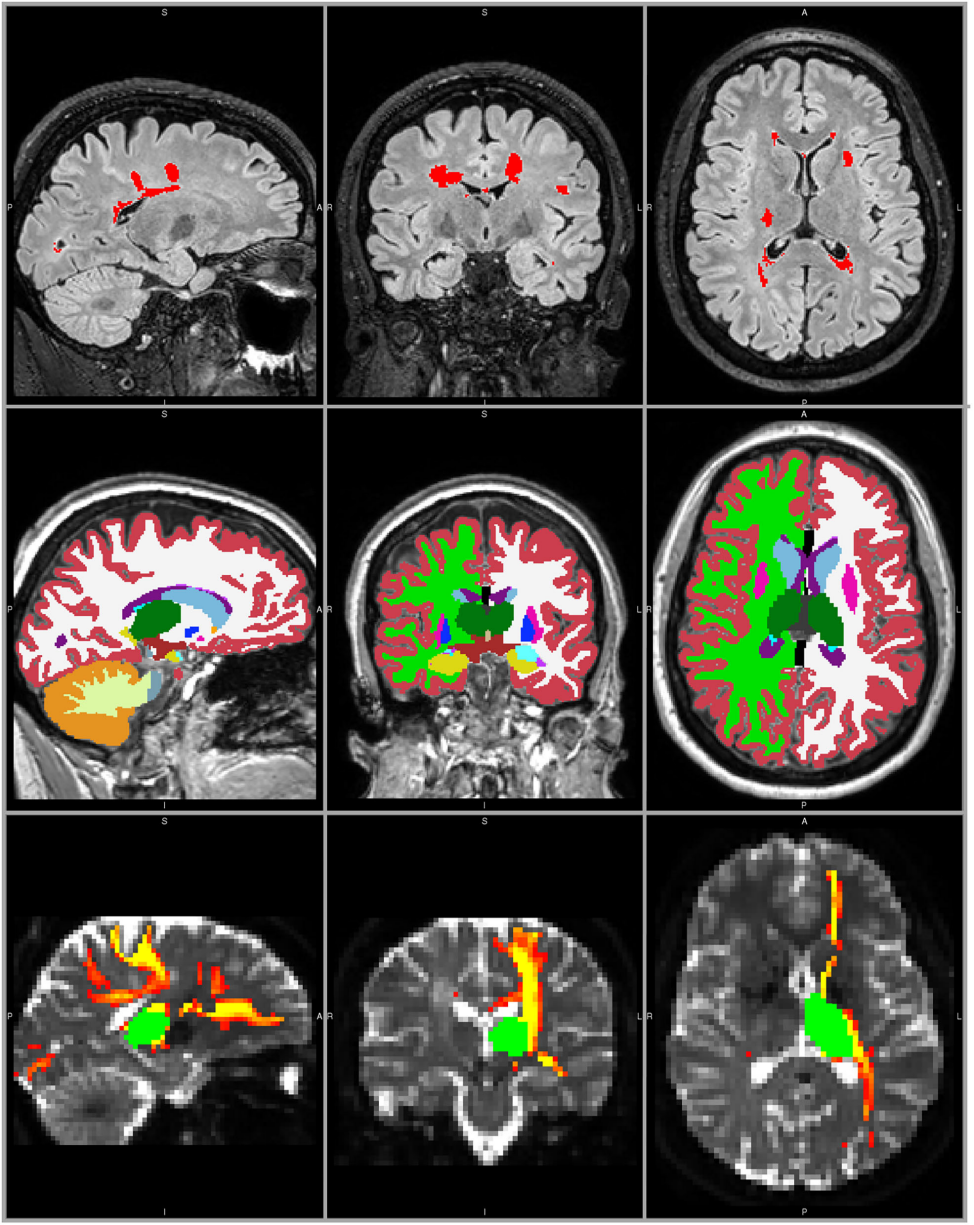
MRI processing example in a 28 year old female with RRMS. Top panels: T2-FLAIR weighted image with LST LPA lesion segmentation (red). Middle panels: T1-weighted lesion filled image with FreeSurfer segmentation, including left and right thalamus (dark green). Bottom panels: non-diffusion weighted image with the registered left thalamus (green) and the probabilistic connectivity distribution map of the corresponding thalamocortical tracts (red-yellow).
